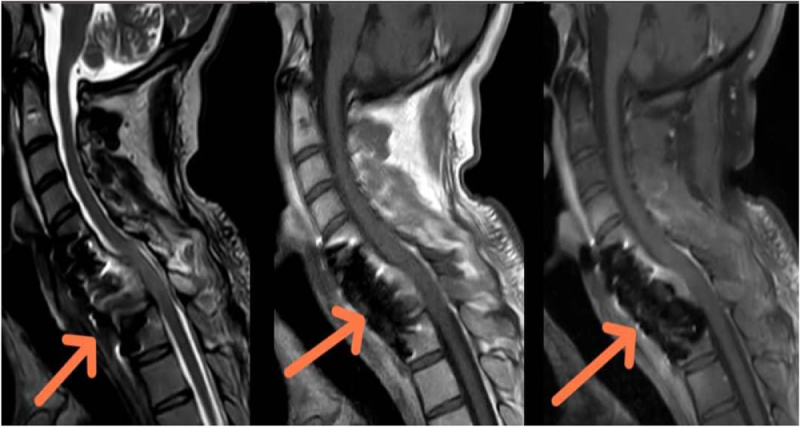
Postoperative MRI shows the artifact from the fixation device.
